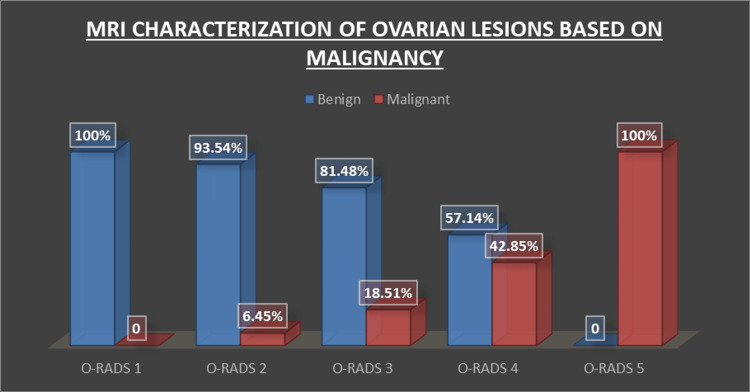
MRI characterization of ovarian lesions based on malignancy O-RADS: Ovarian-Adnexal Reporting and Data System
